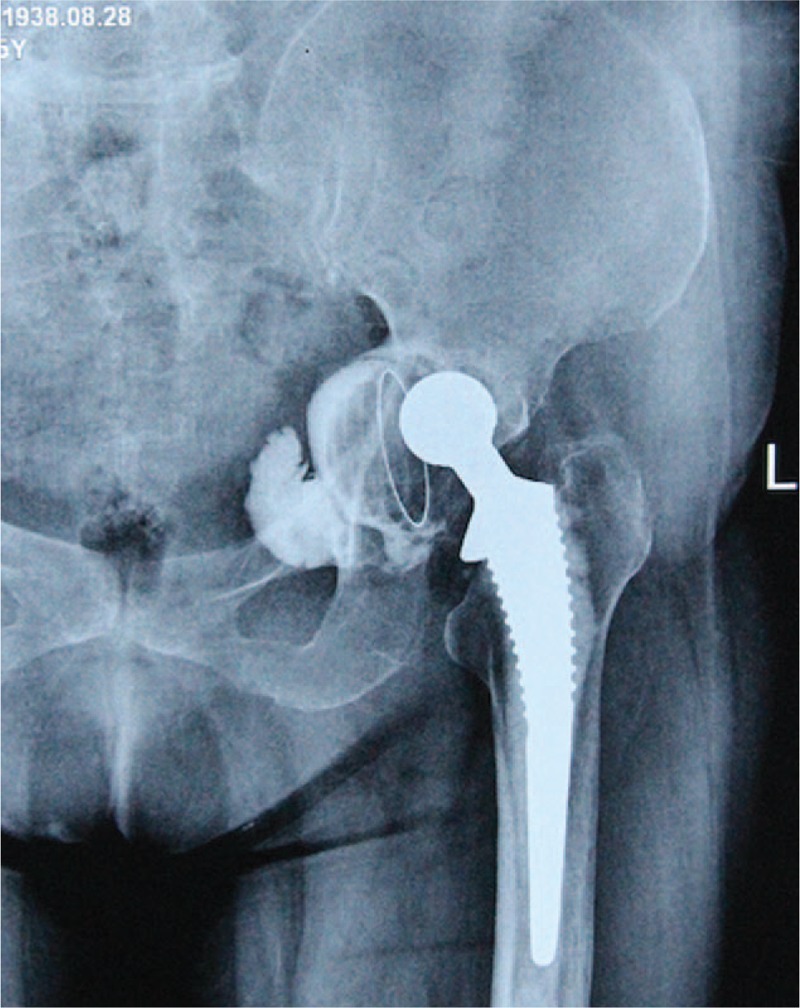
Preoperative anteroposterior radiographs of a 75-year-old female revealing a fixed but malpositioned acetabular component (cemented).
